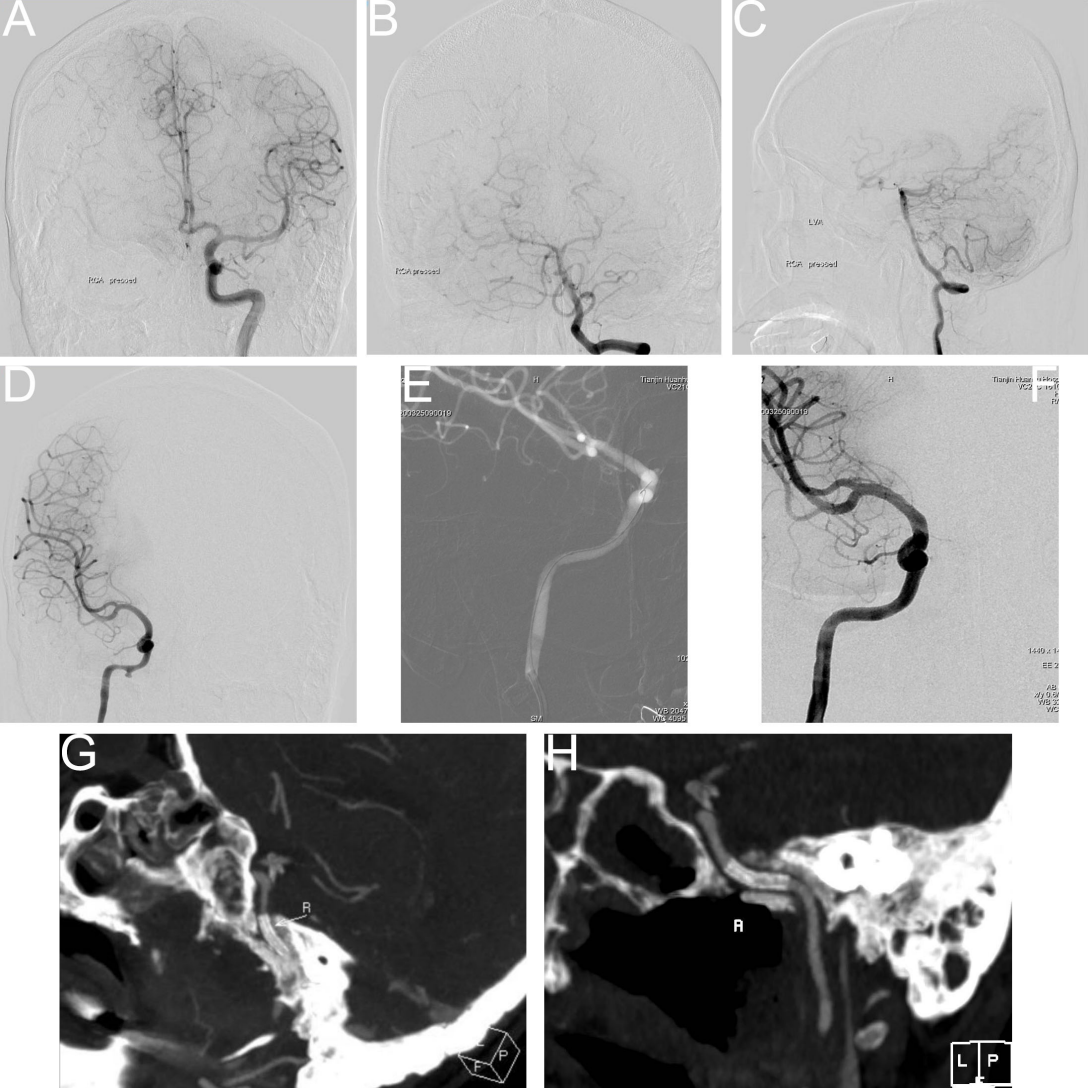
Balloon occlusion test (BOT) procedure steps. (A) Following balloon occlusion of the right internal carotid artery, insufficient compensatory blood flow was observed through the anterior communicating artery. (B) Balloon occlusion of the right internal carotid artery demonstrated limited compensatory blood flow through the posterior communicating artery. (C, D) Pseudoaneurysm identified at the ruptured segment of the right internal carotid artery (prior to stent implantation). (E) Intraoperative image during covered stent placement at the ruptured segment of the right internal carotid artery. (F) Post-stent implantation angiography showed unobstructed blood flow through the treated segment. (G, H) Computed tomography angiography (CTA) confirmed appropriate positioning of the covered stent.
